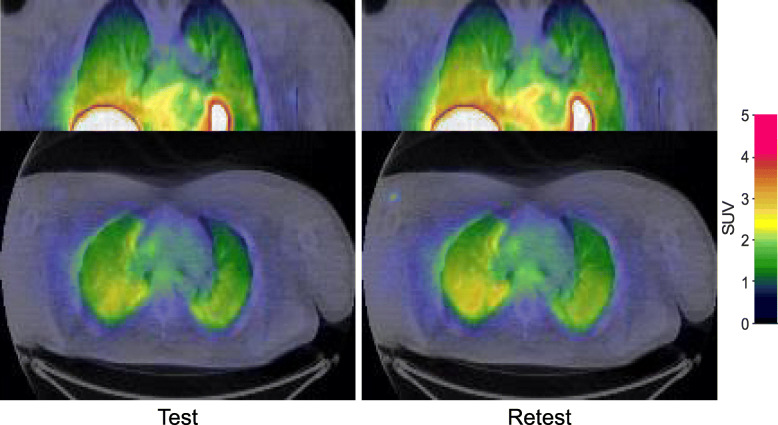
Coronal and axial summation PET images (0–93 min) in thorax after intravenous injection of the radioligand [^11^C]VC-002 in healthy subject #1. Coloured PET images in standardized uptake value units (SUV) are overlaid on structural CT images in grey scale
